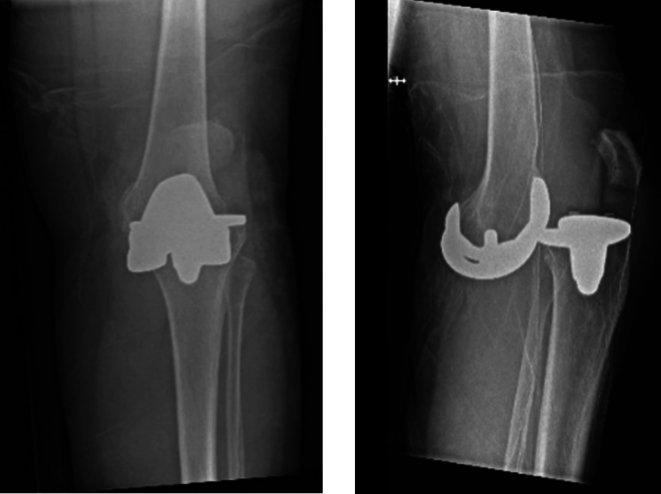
Case 1: conventional radiography images at time of presentation.
